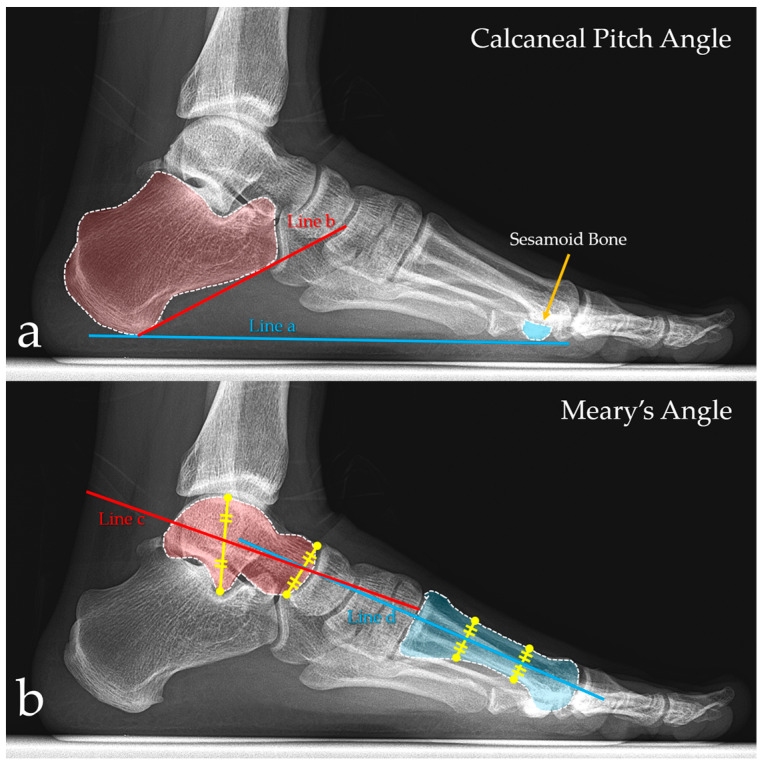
Radiographic assessment of the medial longitudinal arch on weight-bearing lateral foot radiographs. (a) Calcaneal pitch angle measurement: the angle between the floor line (Line a) and the line along the inferior surface of the calcaneus (Line b). The sesamoid bone is shown for orientation. (b) Meary’s angle (talo–first metatarsal angle) measurement: the angle between the longitudinal axis of the talus (Line c) and the longitudinal axis of the first metatarsal (Line d). Yellow lines are used to locate the longitudinal axis of the talus and the first metatarsal bone.
